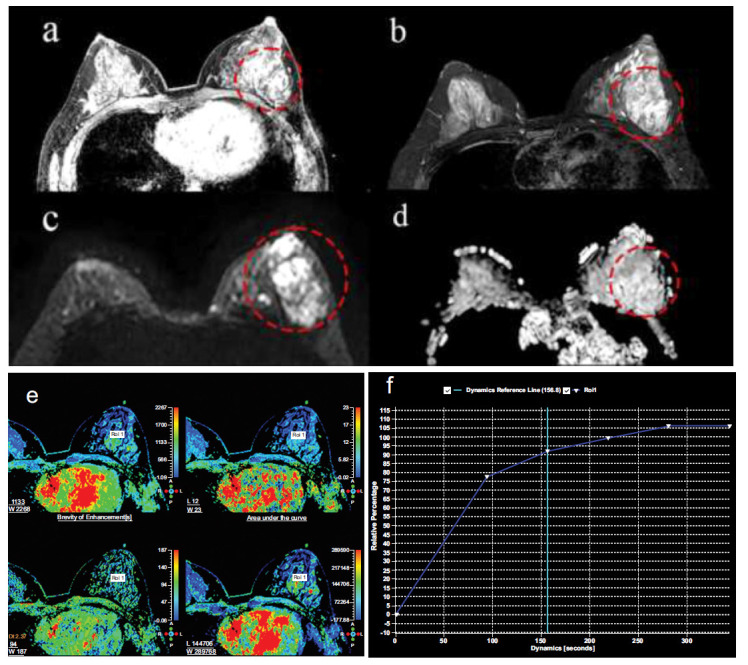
Results of the multi-parameter MRI examination of the patient's breast. (a, b) The lesion in the upper outer quadrant of the left breast showed non-mass enhancement, with a size of approximately 21 × 24mm, blurred edges, irregular shapes, lobulated edges, and burrs. (c) Diffusion-weighted imaging (DWI): The mass area shows a high signal. (d) Apparent diffusion coefficient (ADC): The mass shows a high signal, and a low signal can be observed at the edge. (e) Pseudo-colour parameter map of the left breast. (f) The mass area's time-intensity curve (TIC) shows a rapid-rising plateau type. (The area shown in the red circle is the lesion area).
